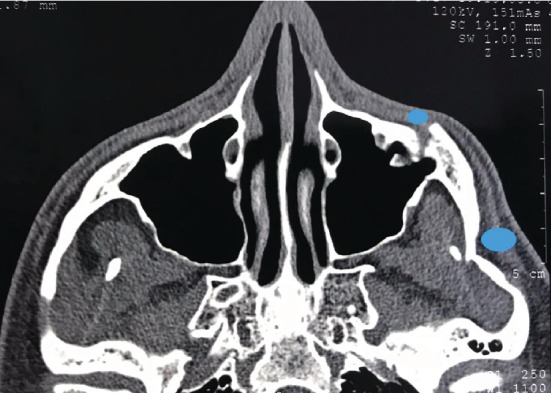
CT scan showing left displaced malar fracture. Blue dots point areas where HA was injected deep to the bone.
